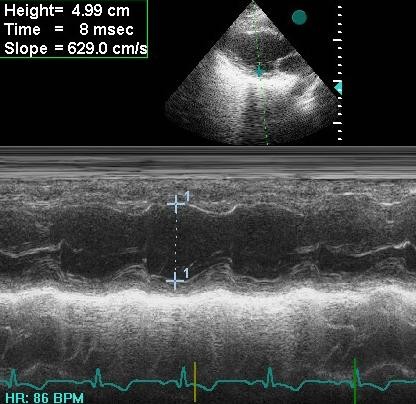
Minor-axis dimension is overestimated due to tangential measurement in M-mode echocardiography (4.99 cm).
